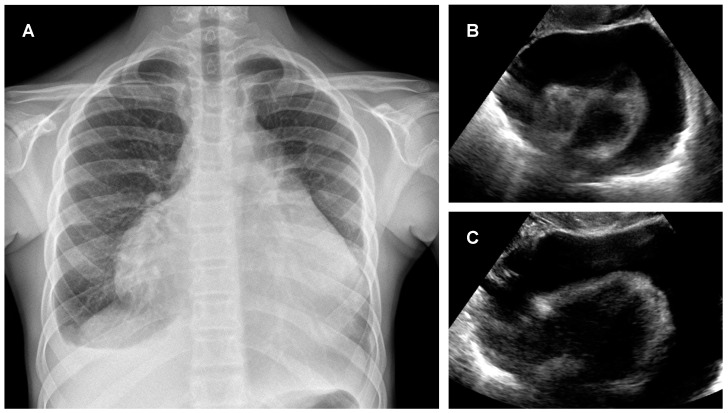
Massive pericardial effusion in a 14-year-old girl. A chest X-ray shows an abnormal cardiac silhouette with an increased cardiothoracic ratio (0.8) and bilateral pleural effusion (A). Echocardiography shows massive pericardial effusion with “swinging heart” signs (B,C).
